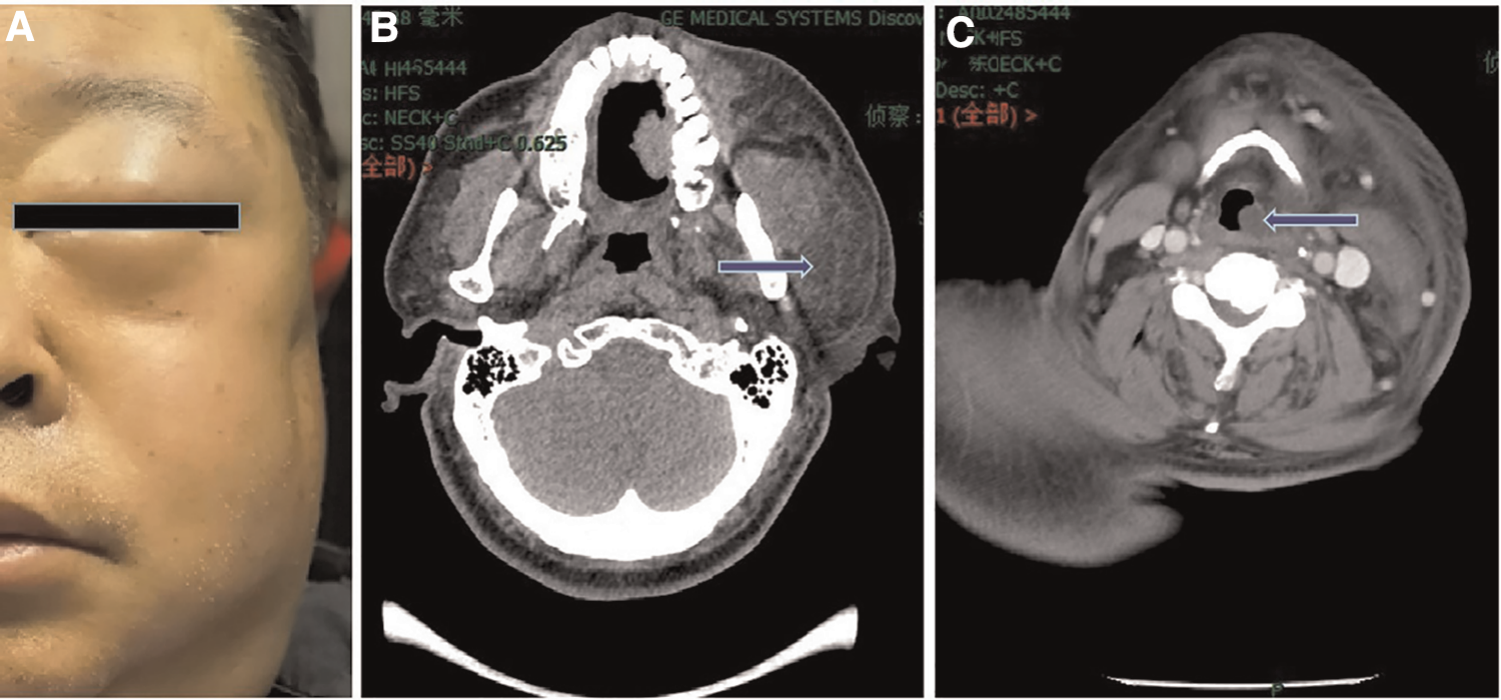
Clinical appearance and imaging findings of the patient. (A) Photograph of the patient showing swelling of the left eyelid and parotid gland area. (B) Enhanced CT image showing significant enlargement of the left submandibular soft tissue and parotid gland (arrow). (C) Enhanced CT image showing the involvement of the left parapharyngeal space and airway stenosis (arrow).
